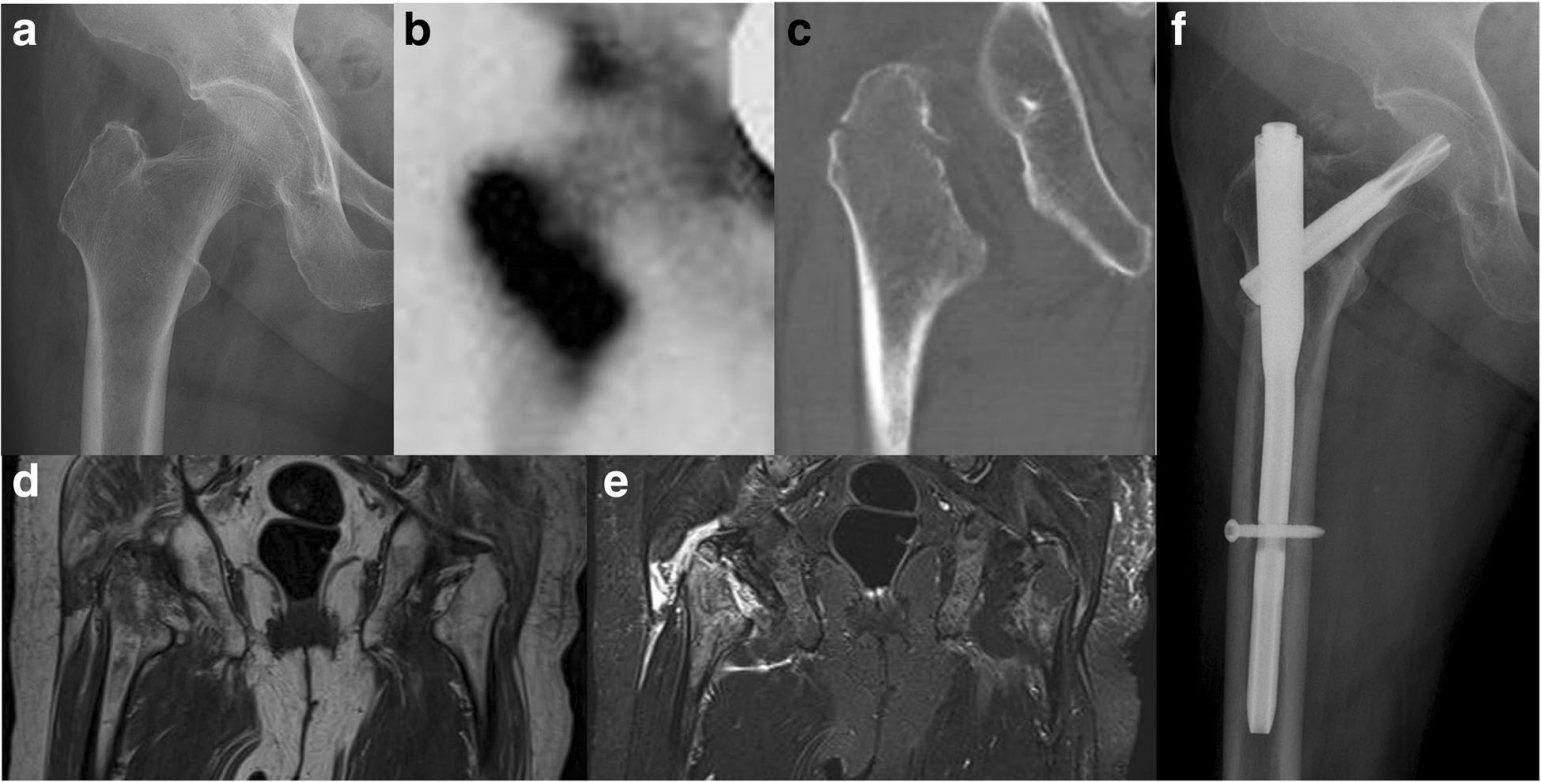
Case of a 73-year-old woman presented at the emergency department with right hip pain after slipping in a convalescent hospital. a A plain radiograph of the right hip shows an isolated greater trochanter fracture. b A bone scan performed 6 days after the trauma reveals that the fracture extended into the intertrochanteric region. c A computed tomography scan shows an isolated greater trochanteric fracture without intertrochanteric extent. d, e Magnetic resonance imaging shows an intertrochanteric fracture that crosses the midline to the medial cortex. f Internal fixation was performed using proximal femoral nail antirotation
